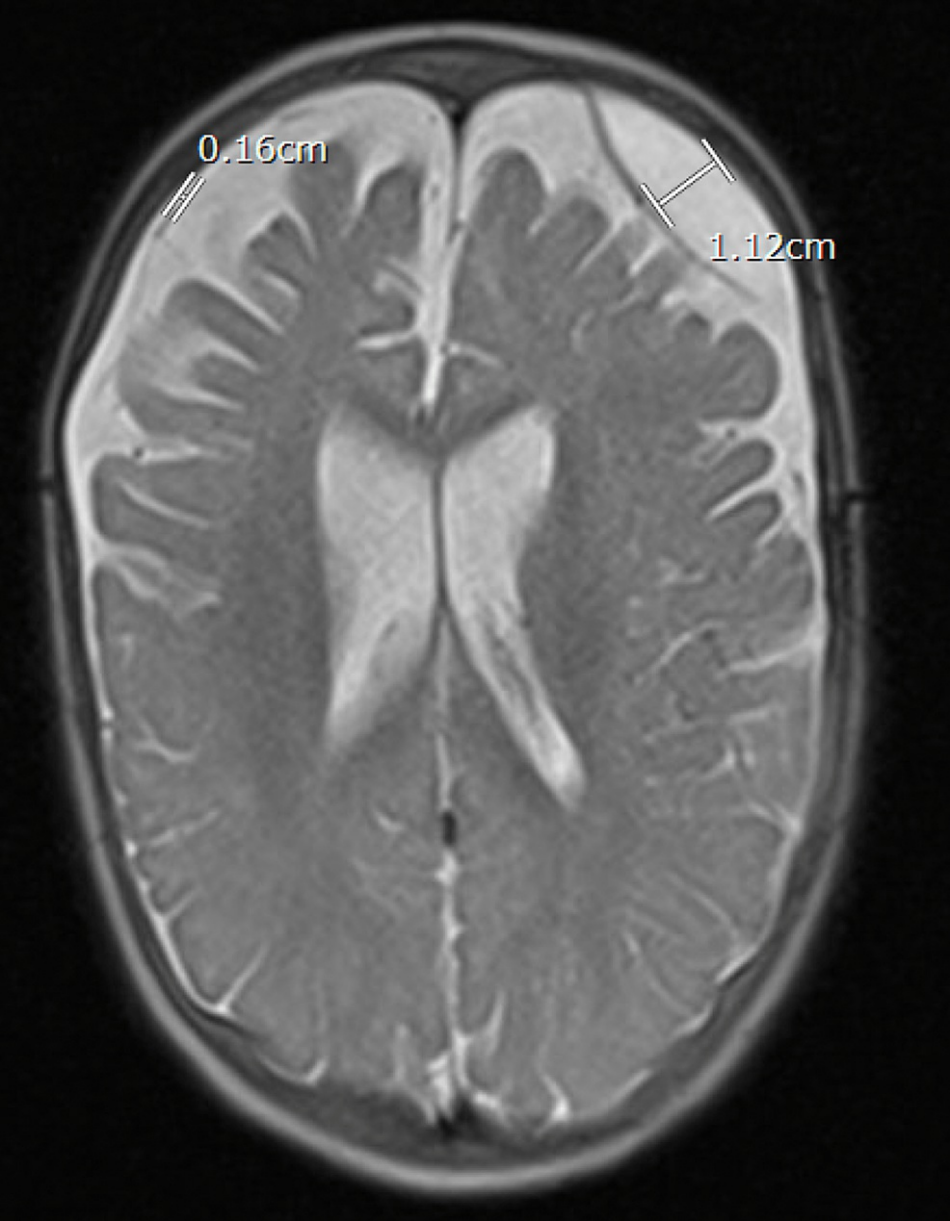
MRI of the brain T2 axial view showing bilateral subdural hygroma.
